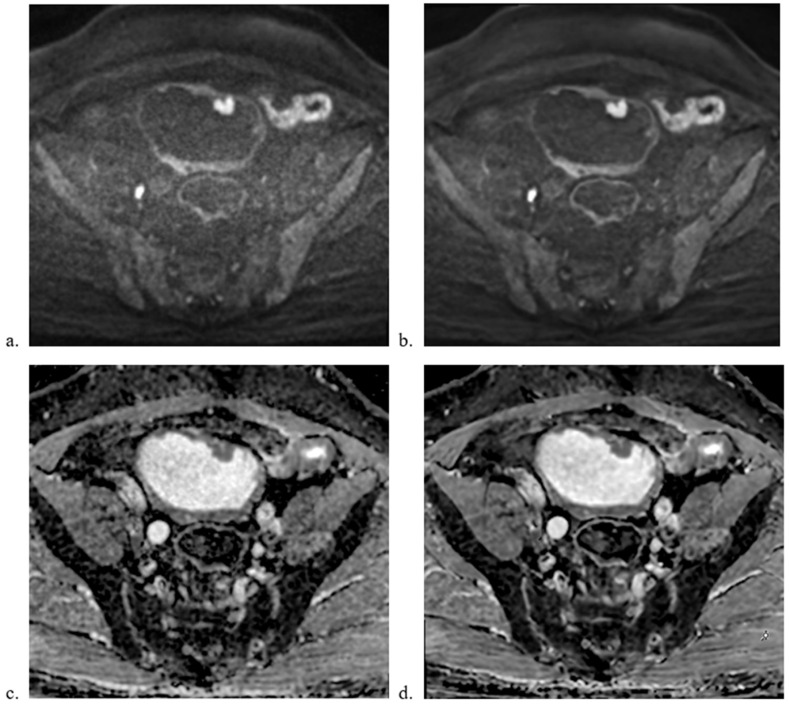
Comparison of MUSE-DWI and ARDL MUSE-DWI datasets. A 65-year-old male underwent bladder MRI. Multiple masses were seen including the two masses at the anterior wall shown in the images. Radical cystectomy confirmed multiple non-invasive papillary urothelial carcinoma. Axial images showing (a) MUSE-DWI, (b) DL MUSE-DWI, (c) ADC map generated from MUSE-DWI, and (d) ADC map generated from DL MUSE-DWI. The ARDL dataset showed better sharpness and lesion conspicuity.
